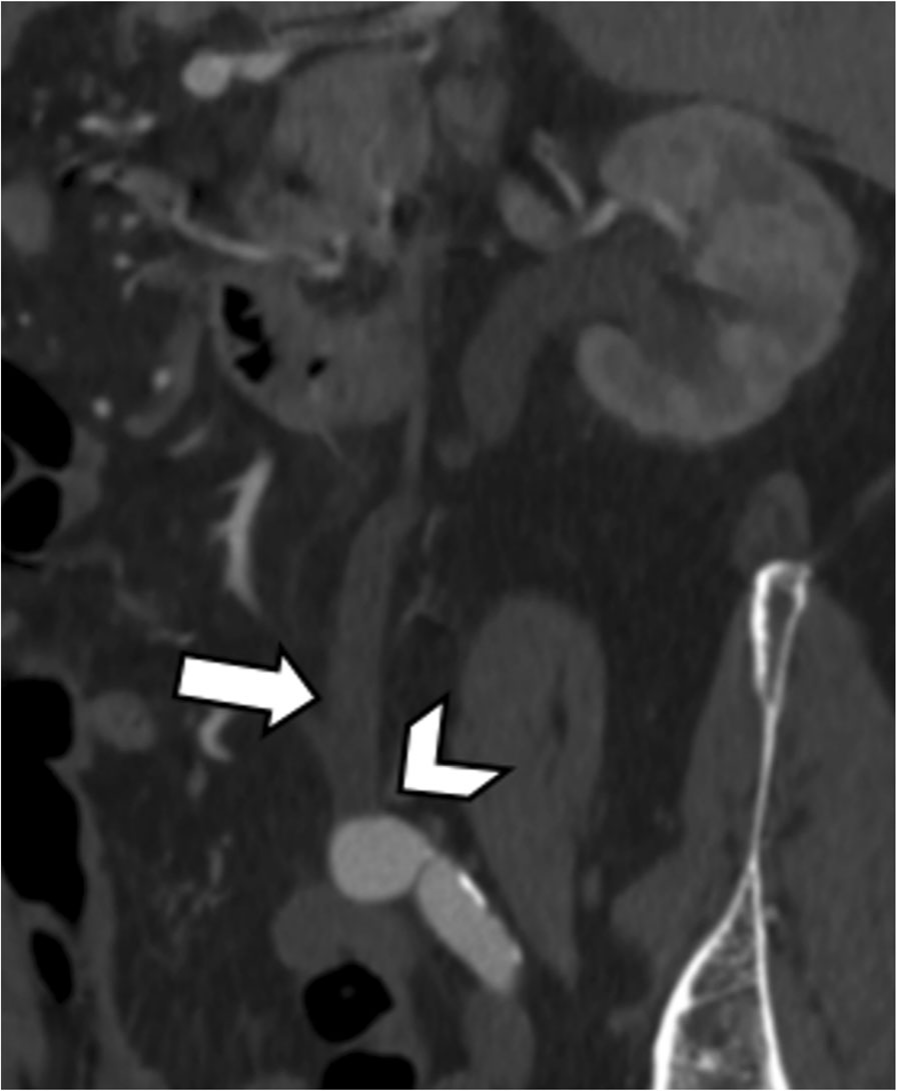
An 83-year-old man with ureteral vascular compression. Sagittal CT image on arterial phase shows right hydroureteronephrosis (arrow) secondary to compression by a saccular aneurysm (arrowhead) of the adjacent right common iliac artery
